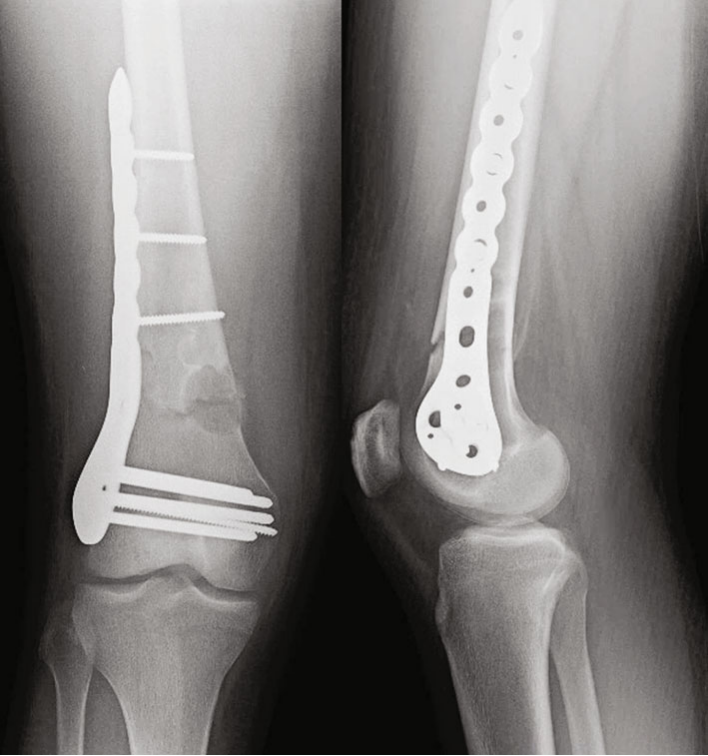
AP and lateral plain radiographs taken in clinic approximately 1 month postoperatively, demonstrating healing fracture.
